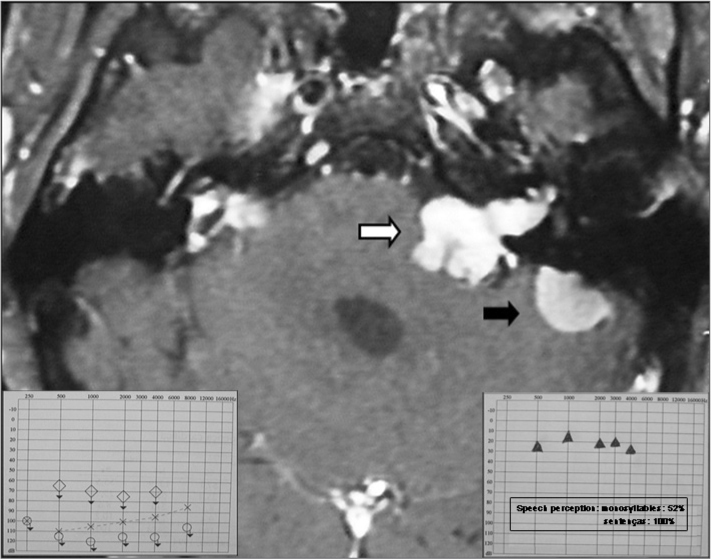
Magnetic resonance imaging (T1 contrast) showing a vestibular schwannoma to the left (white arrow) associated with a meningioma on the medial aspect of the left petrous bone (black arrow). Note also small manipulation remains in the right pontocerebellar angle. On the lower left detail, see preoperative audiometry. On the lower right detail see audiometry 6 months after the cochlear implant.
